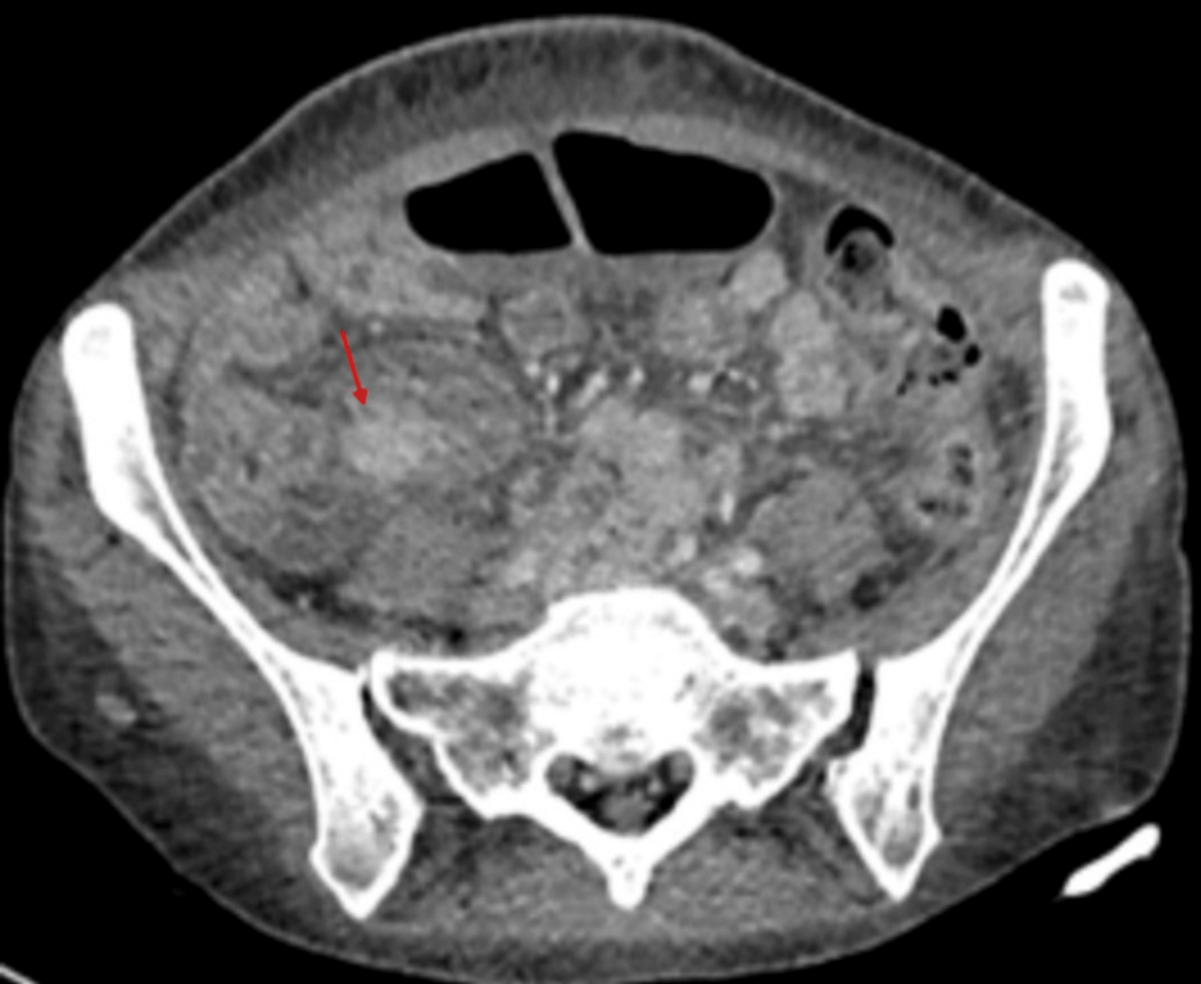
Repeat CT of the thorax/abdomen/pelvis on 7 February 2025 showing stable retroperitoneal fibrosis and a mild increase in size of the enhancing lesion at the ileocecal junction (red arrow).
